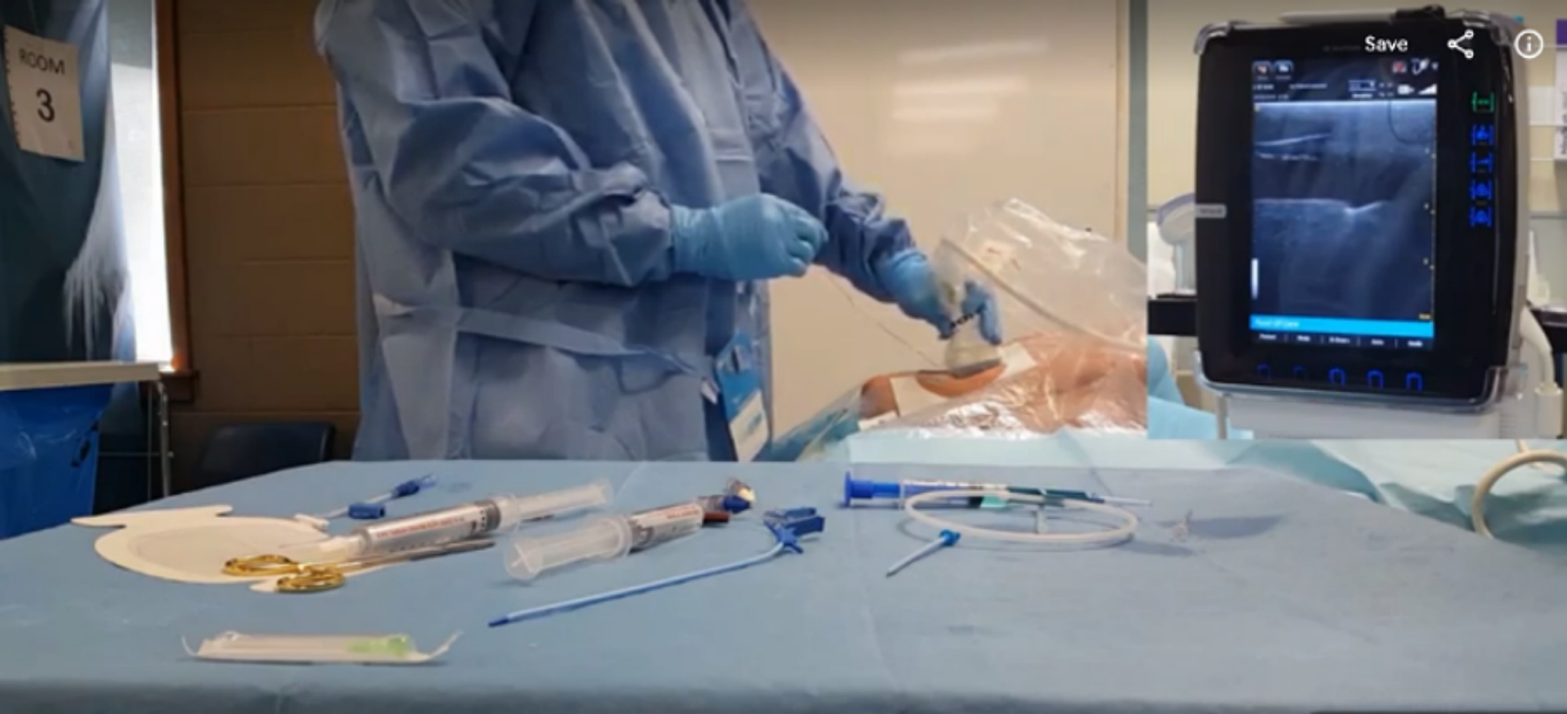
A sample of the video created from a participant’s test session, showing their performance with the video of the ultrasound matched in a picture-in-picture view
